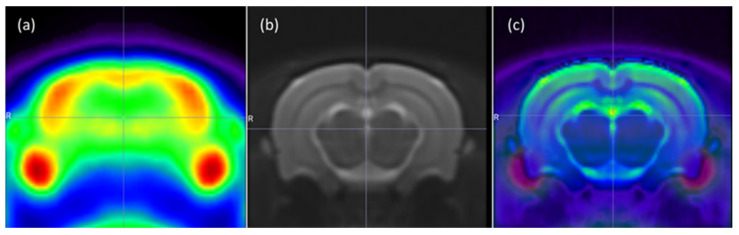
Coronal section of: (a) 18F-FDG PET brain template, on a Rainbow Color Scale; (b) reference MRI template, on a Gray Color Scale; and (c) fused 18F-FDG PET/MRI. Letter “R” represents the right side of the image.
